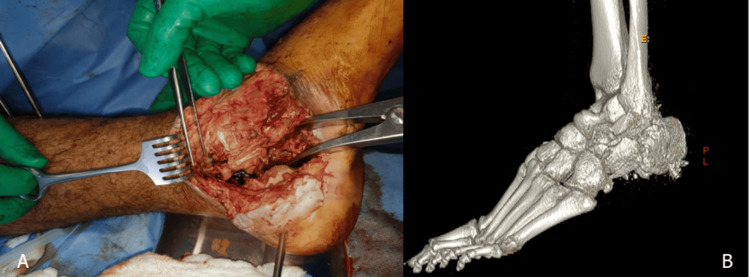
(A) Intraoperative and (B) 3D CT image of medial aspect of the left foot showing calcaneal defect.
